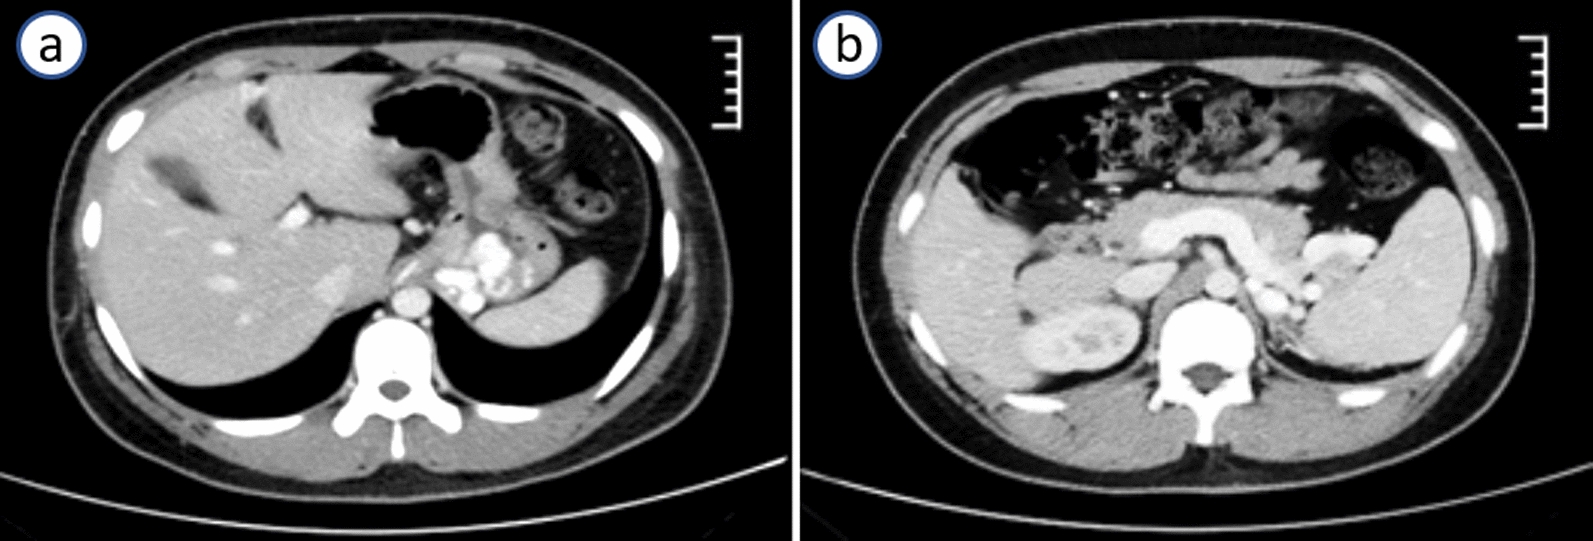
Enhanced computed tomography scan of the abdomen showing varicose veins in the lower esophagus and gastric fundus (a), dilation of main portal vein, and splenomegaly (b)
